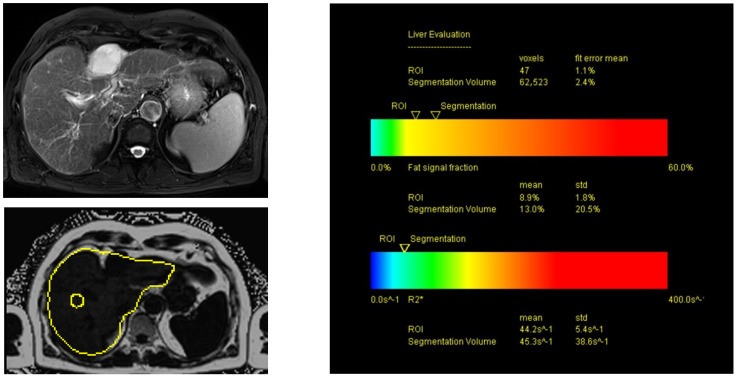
MRI T2-weighted (left upper image, T2 BLADE (fs)) and PDFF mapping (left lower image) in axial view in a patient with hepatic steatosis and initial fibrosis. The fat signal fraction inside the manually placed ROI (yellow circle in left lower image) showed a mean of 8.9%, consistent with mild to moderate hepatic steatosis.
